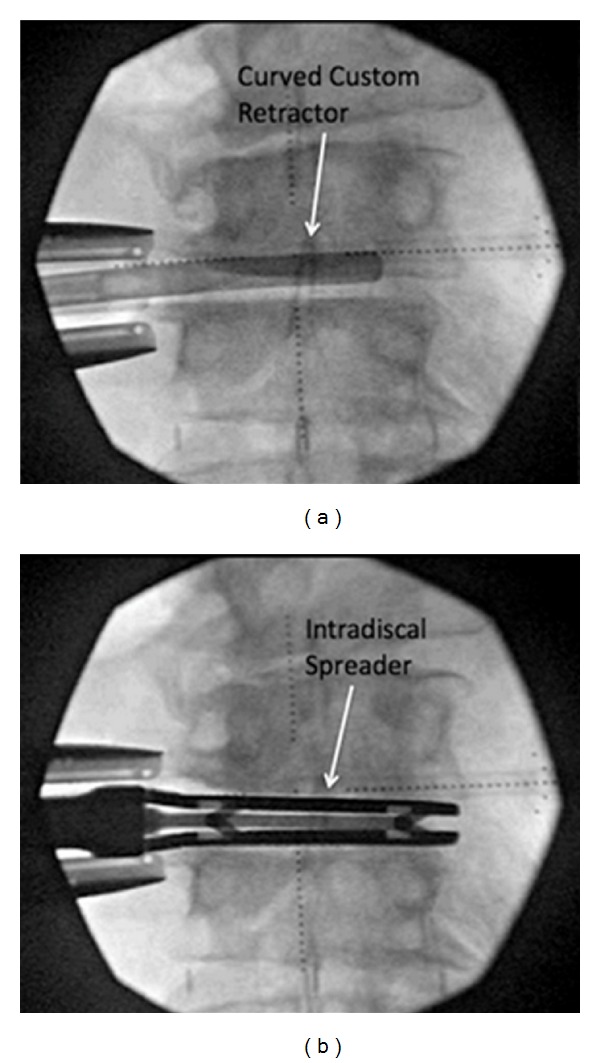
Intraoperative anterior-posterior radiographs demonstrating the curved retractor anterior to the disc space during dissection of the sympathetic plexus off the great vessels (a), and the intradiscal spreader in the disc space used to break the contralateral ALL remnant rather than blindly incising (b).
